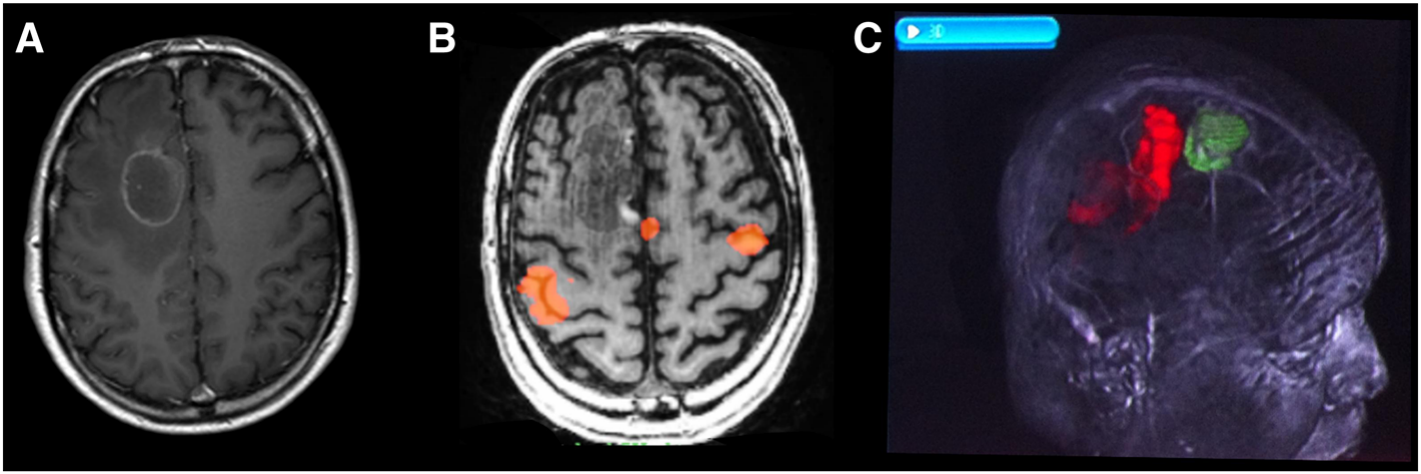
A patient known to have breast cancer with a manually dexterous job presented with intermittent left hand and arm weakness and was found (A) to have a solitary ring enhancing lesion in the premotor area on T1W MRI with gadolinium. (B) functional MRI performing a hand tapping and gripping task determined the location of hand function and this was used as the “seed” region of interest on a DTI scan to produce a representation of the motor tracts. (C) these were used to generate a 3D object and fused with an anatomical planning scan (1 mm slices) using commercially available software (StealthViz™ with StealthDTI™ by Medtronic, running on an S7 workstation) to produce images that were used intraoperatively for image guided resection, avoiding the tracts (shown in red, with tumour rendered in green).
